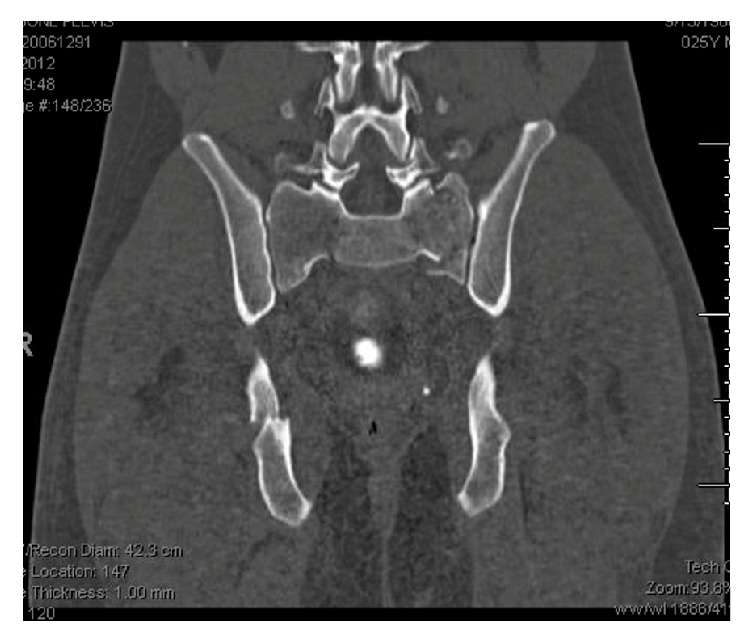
Preoperative coronal CT scan of a patient with spinopelvic dissociation.
